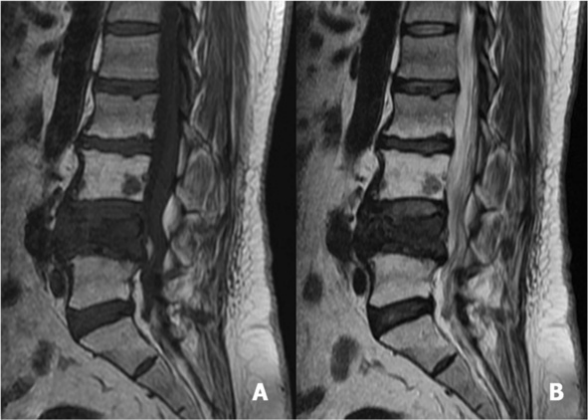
Sagittal T1-weighted (a) and T2-weighted MRI (b) revealed a pathological fracture of L4, with bone marrow oedema and protrusion into the spinal canal. A small lesion at the body of L3, without a significant effect on structural stability, was identified
